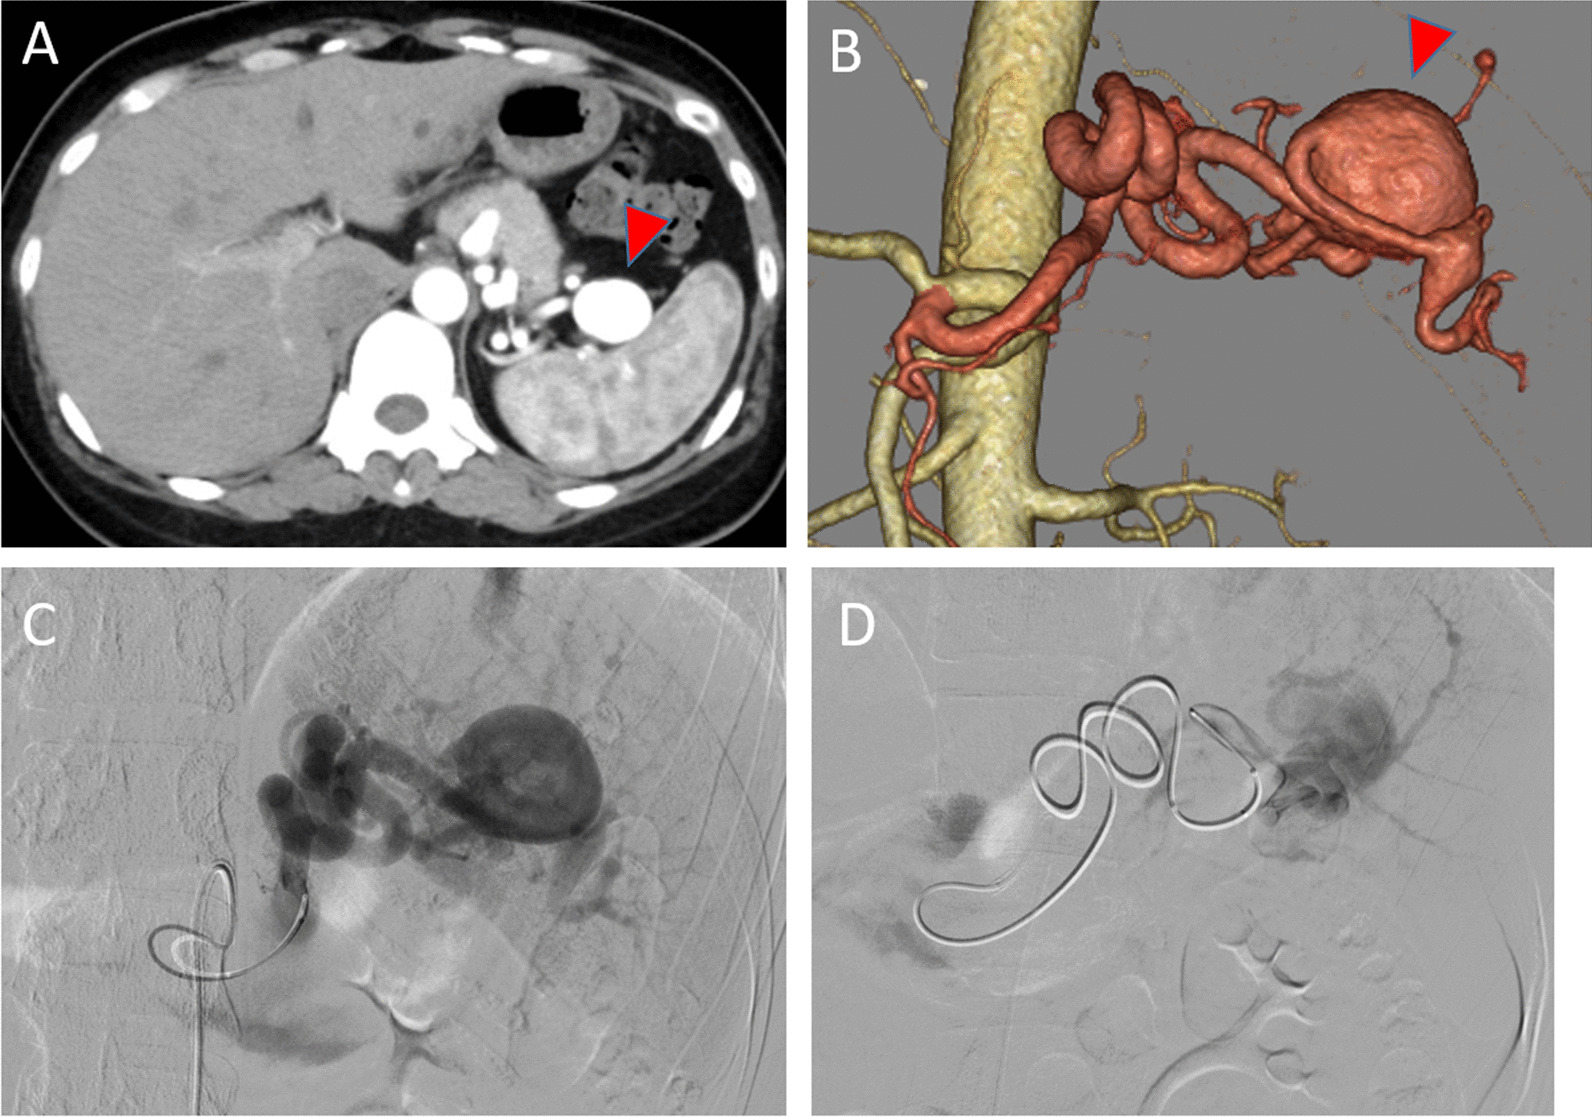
A, B Contrast-enhanced abdominal computed tomography showed a 28-mm splenic artery aneurysm at the splenic hilum (arrowhead). C Angiography showed a splenic artery aneurysm. D Transcatheter arterial embolization was attempted; however, this was difficult due to tortuosity of the splenic artery
